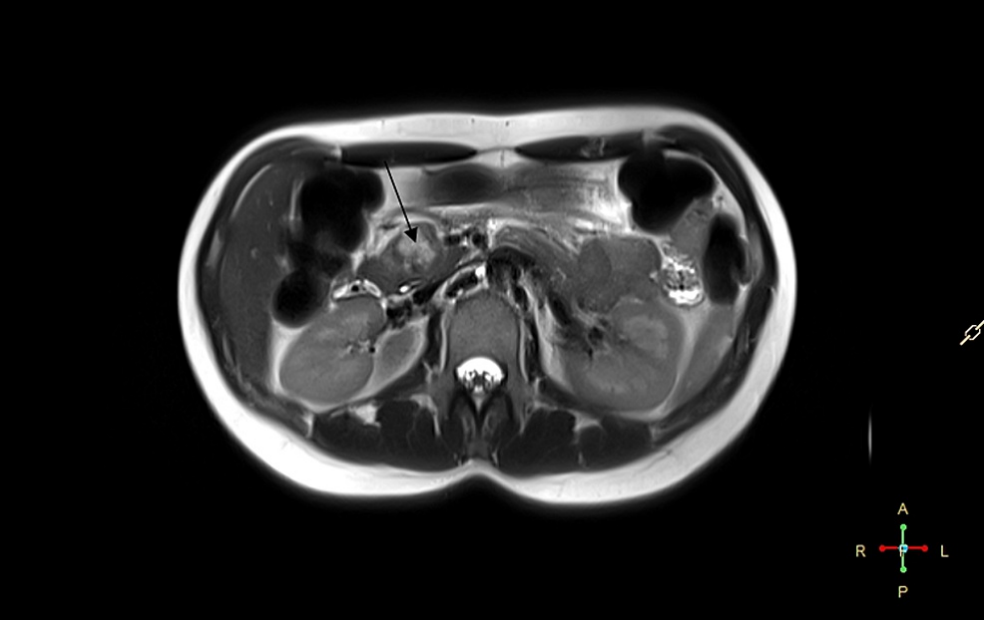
MRI scan arrow pointing the well-described pancreatic tumor
